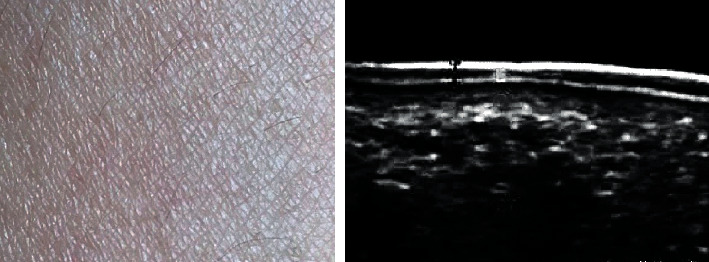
Normal skin and ultrasound images.
